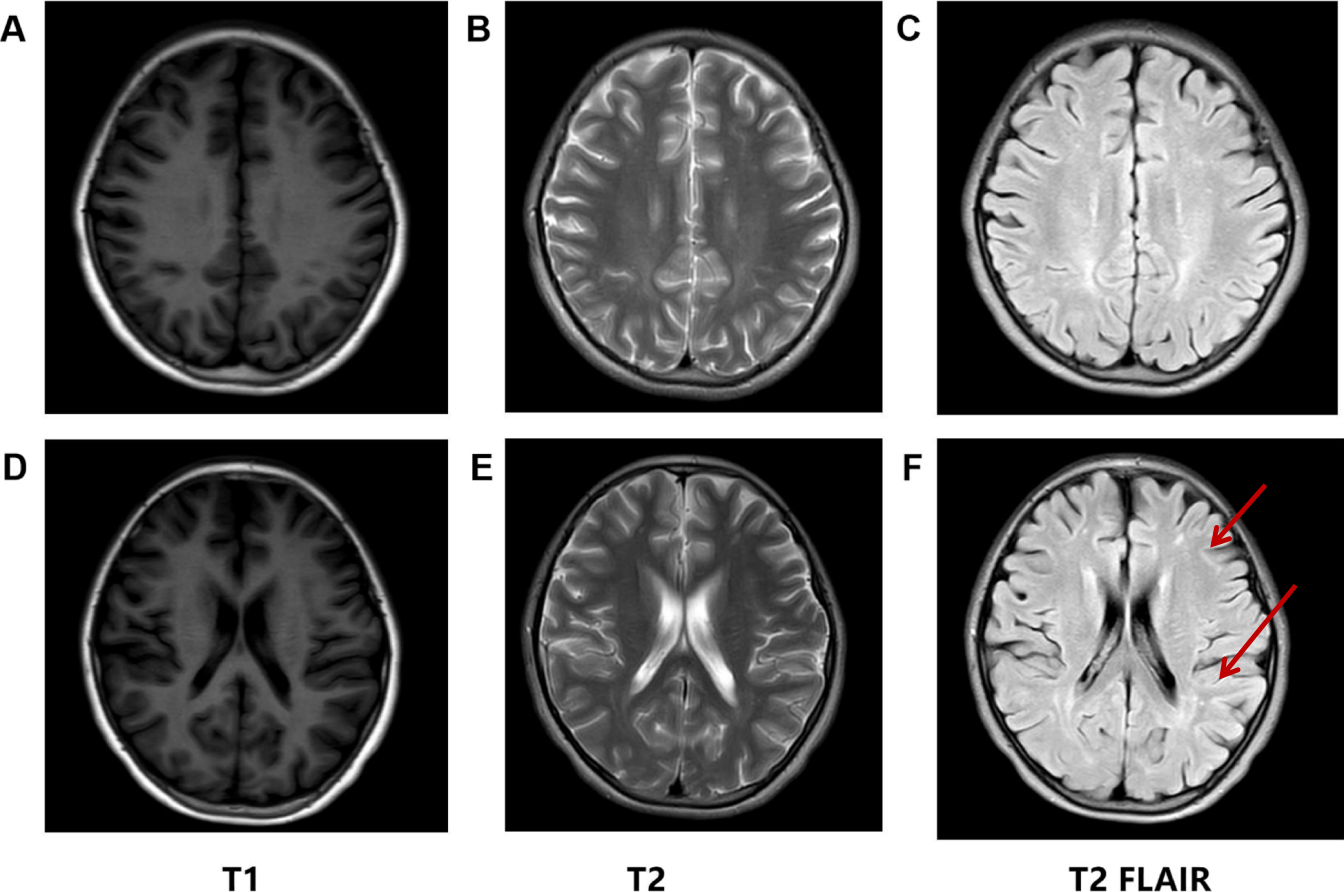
Brain MRI of the patient at the time of the first study. Brain MRI (3.0T) were performed at the Children’s Hospital of Nanjing Medical University. Axial T1 images (A, D), T2 images (B, E), and T2 fluid-attenuated inversion recovery images (C, F). B, E Small lamellar slightly long T2 signal shadow in the white matter of the frontoparietal brain on both sides, and high signal intensity on T2 FLAIR (C, F) images involving the white frontoparietal white matter on both sides (red arrows)
